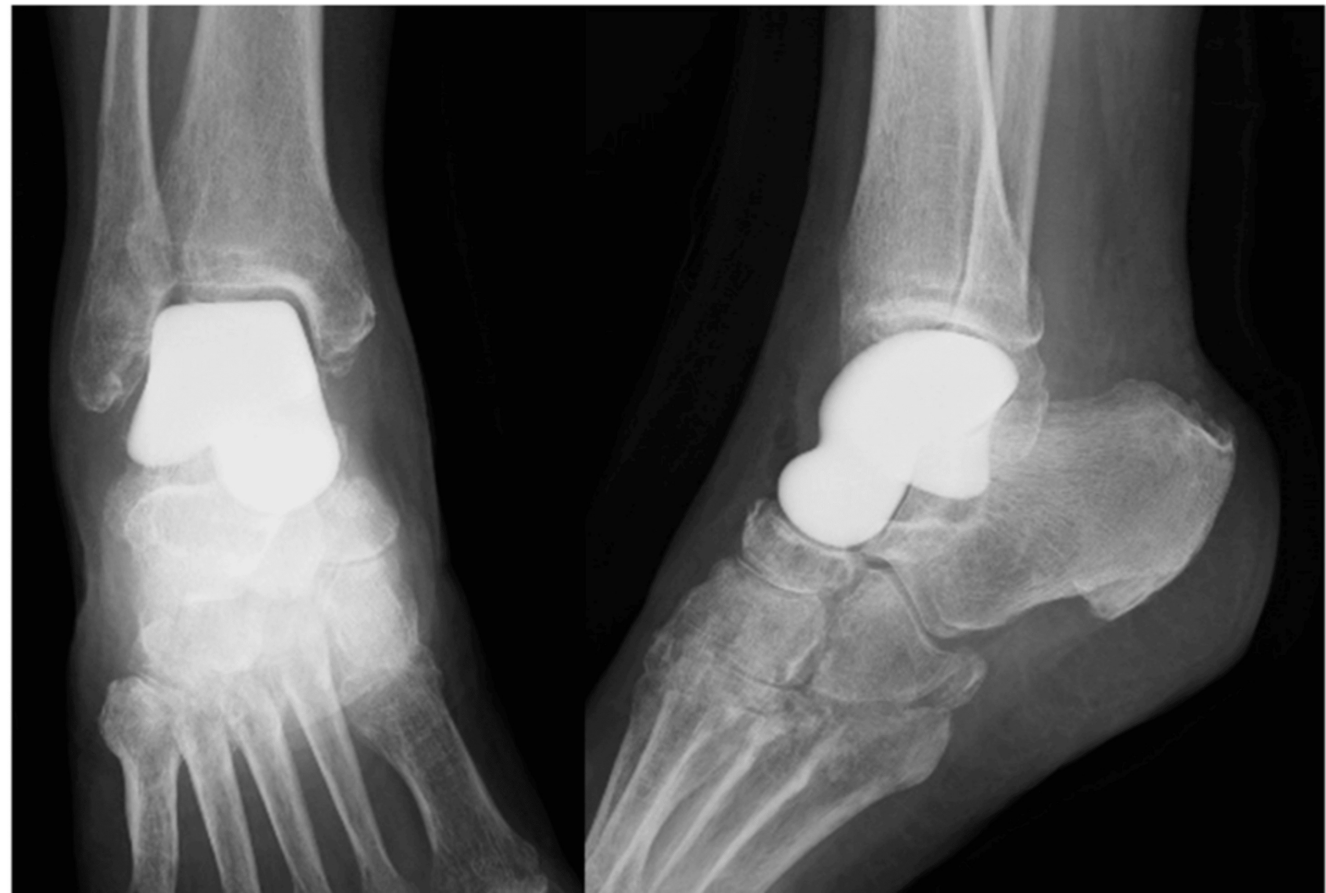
An X-ray image after wide excision with custom-made alumina ceramic total talar prosthesis.
